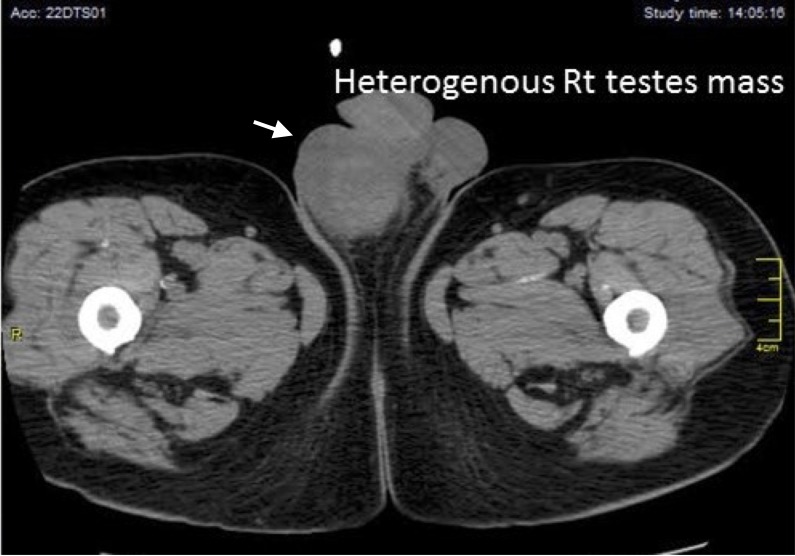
Figure showing the computed tomography (CT) scan of right heterogeneous testicular mass with hyperdense areas
